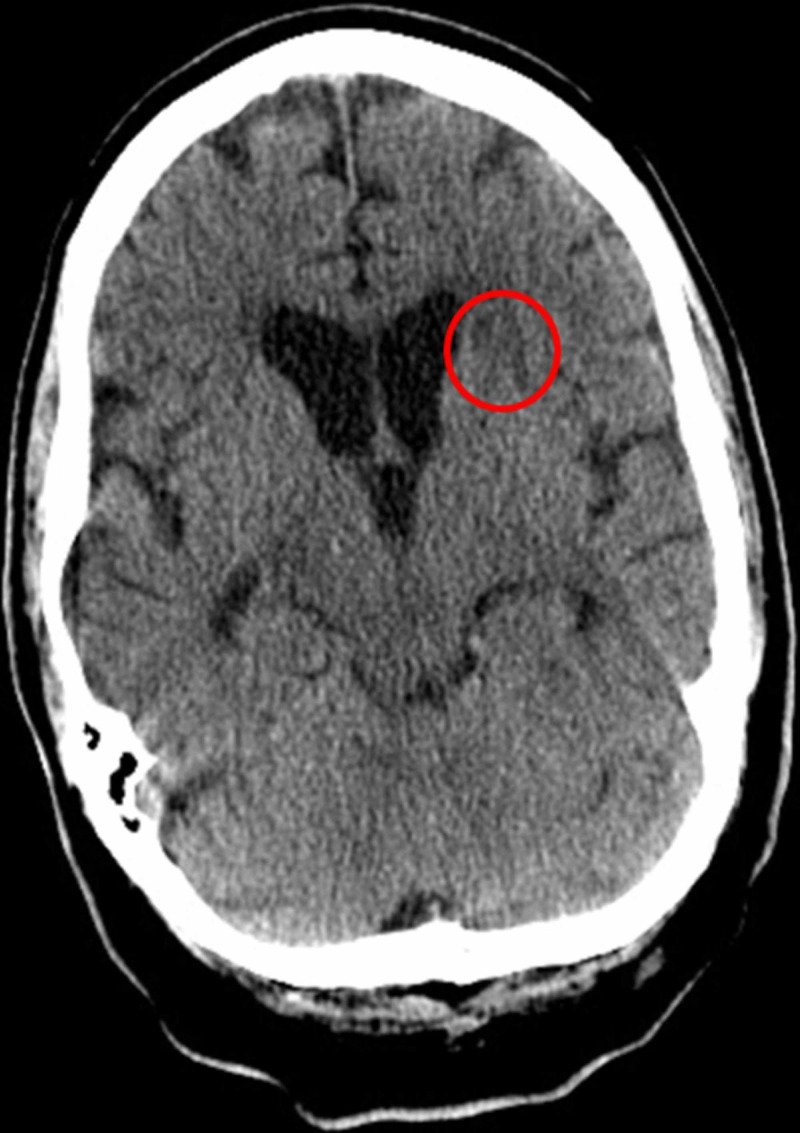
Follow-Up CT of the Brain Demonstrating Resolution of a Periventricular Abscess Follow-up axial CT of the brain shows resolution of a previously demonstrated periventricular abscess on MRI. There is a remnant of periventricular edema where the abscess was localized, shown by hypoattenuation (red circle).
